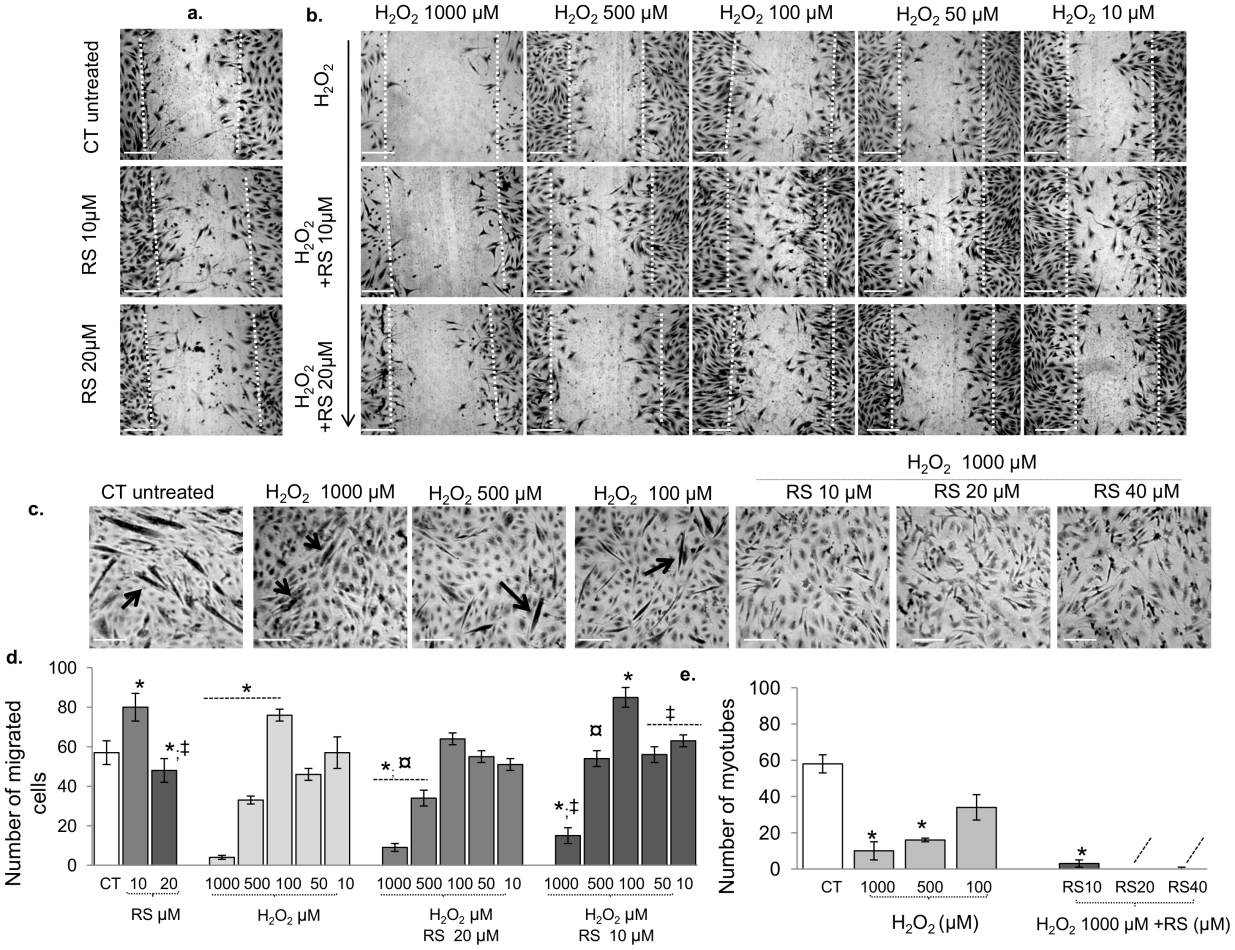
Resveratrol (10 µM) prevented the deleterious effect of H_2_O_2_ on cell migration but not cell fusion. (a) Phase contrast images showing the effect of RS alone (10 and 20 µM) on cell migration (24 h). (b, c) Phase contrast images showing the effects of different pre-conditioning resveratrol (RS) concentrations and H_2_O_2_ on cell migration (b) and fusion (c). The number of migrated cells and myotubes in the different conditions are summarized in (d) and (e), respectively. (b and graph in d) show that the effects of 24 h H_2_O_2_ on cell migration were dose-dependent. High doses of H_2_O_2_ (500–1000 µM) blocked cell motility, while it was increased with 100 µM H_2_O_2_. (b and graph in d) shows that RS pre-conditioning (10 µM) abolished the deleterious effects of 500 µM, attenuated that of 1000 µM H_2_O_2_ and further enhanced cell migration induced by H_2_O_2_ 100 µM. (c,e) 500 and 1000 µM H_2_O_2_ did block cell fusion, which was not rescued with RS preconditioning. Data are expressed as mean ± s.e.m of biological quadruplicate. *: *P*<0.01 vs. CT; ‡: *P*<0.01 vs. RS 10 µM; ¤: *P*<0.01 vs. RS 20 µM. *P*-value calculated using a two-tailed Student's *t*-test. Bars 20 µm. Original magnification, x 50.
